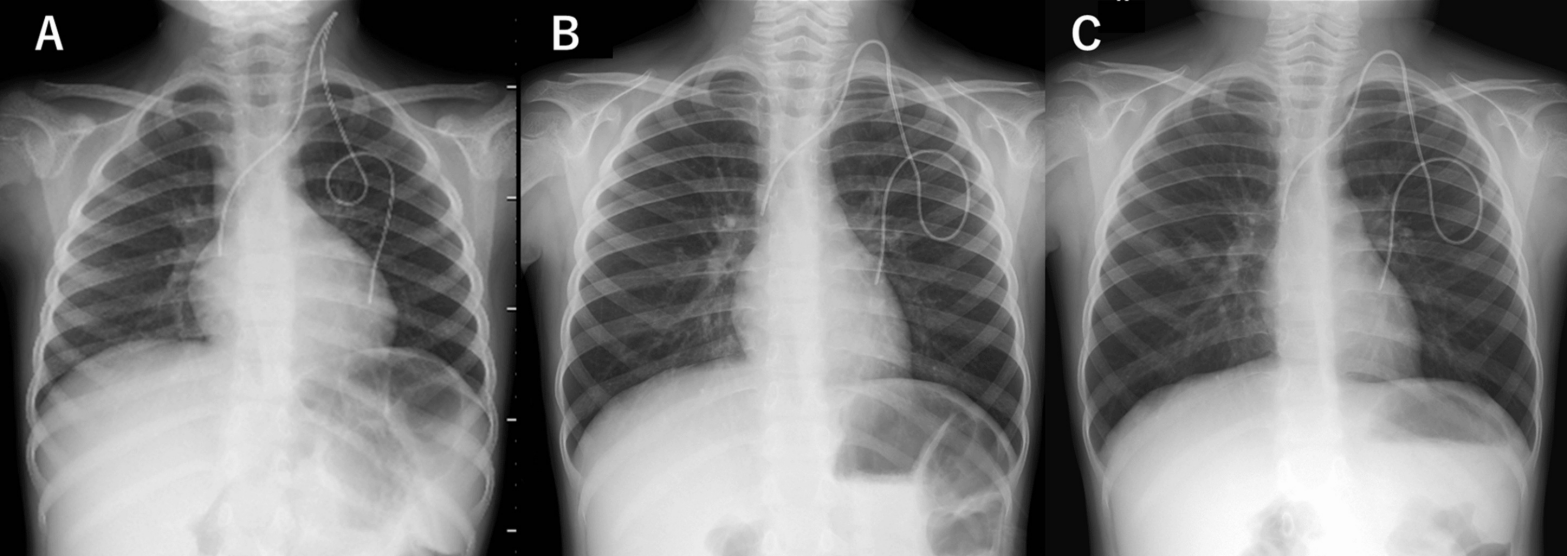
Progressive hyperinflation persisting over six weeks despite treatment with bronchodilators and corticosteroids (A) Chest radiograph prior to onset shows no remarkable hyperinflation. (B) Two weeks after symptom onset, hyperlucency, diaphragmatic flattening, and a barrel-shaped thorax are observed. (C) Six weeks after onset, hyperinflation findings had progressed despite treatment with bronchodilators and corticosteroids
